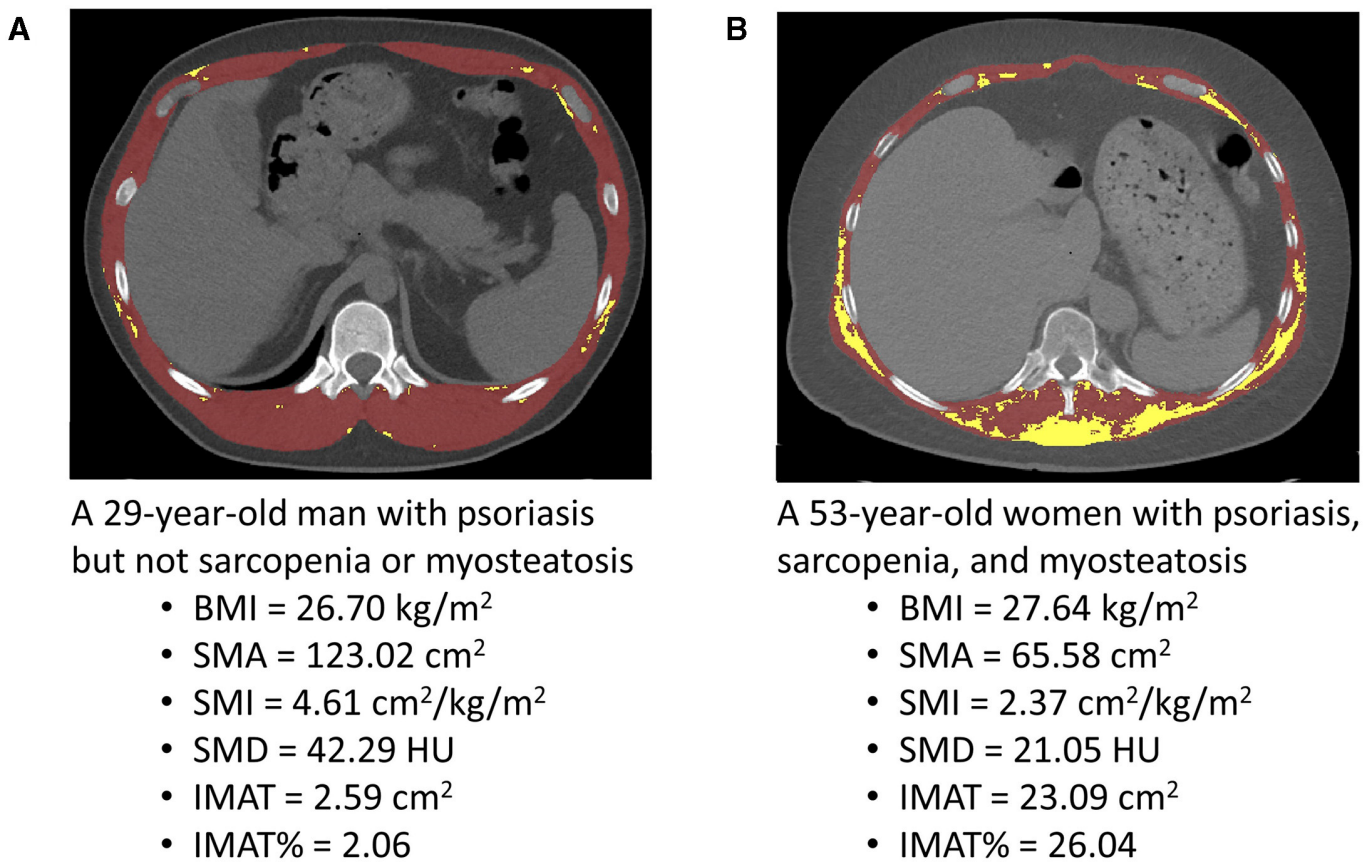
Typical segmented computed tomography (CT) images at the 12th thoracic vertebra level used for the quantification of the skeletal muscle area (SMA), skeletal muscle radiodensity (SMD), and intermuscular adiposity tissue (IMAT) in a young man with psoriasis (A) and a middle-aged woman with psoriasis (B). BMI, body mass index; HU, Hounsfield unit; SMI, skeletal muscle index; IMAT%, percentage of IMAT.
